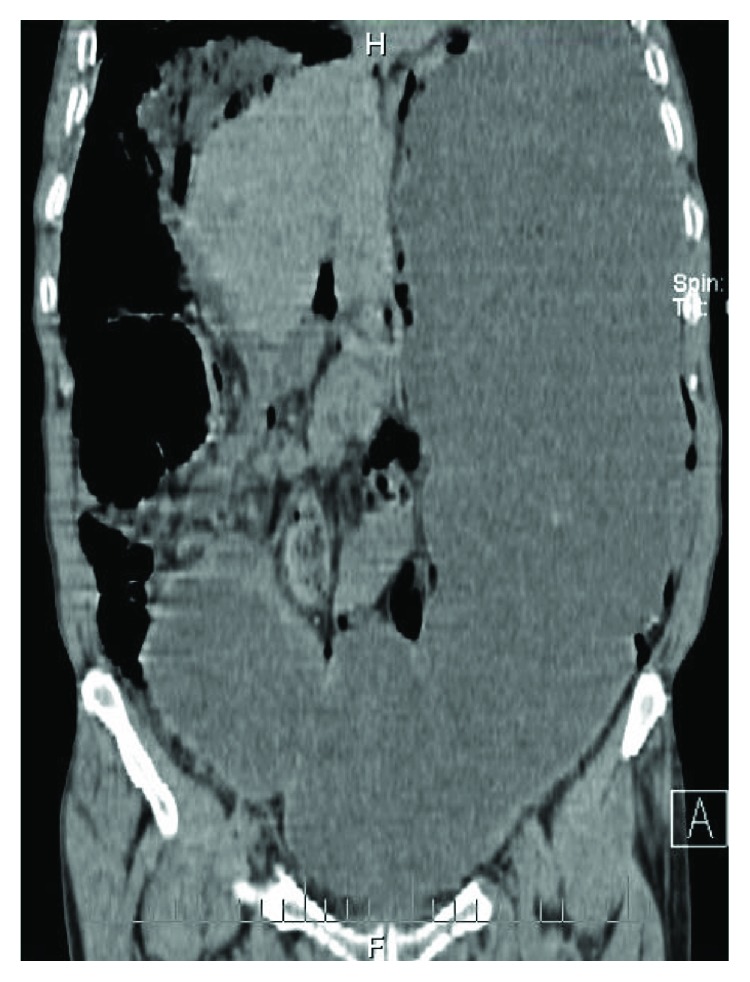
Abdominal CT scan reveals massive gastric distension.
